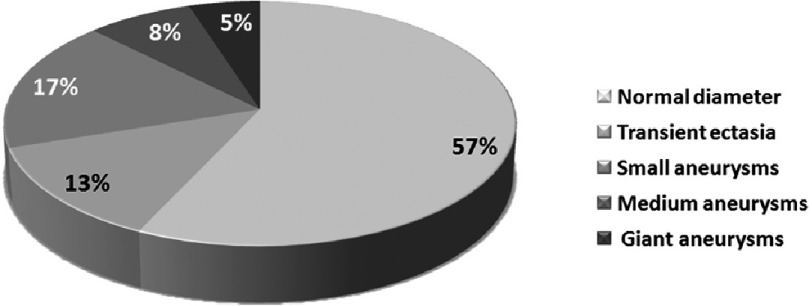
Coronary artery outcomes by echocardiography.
